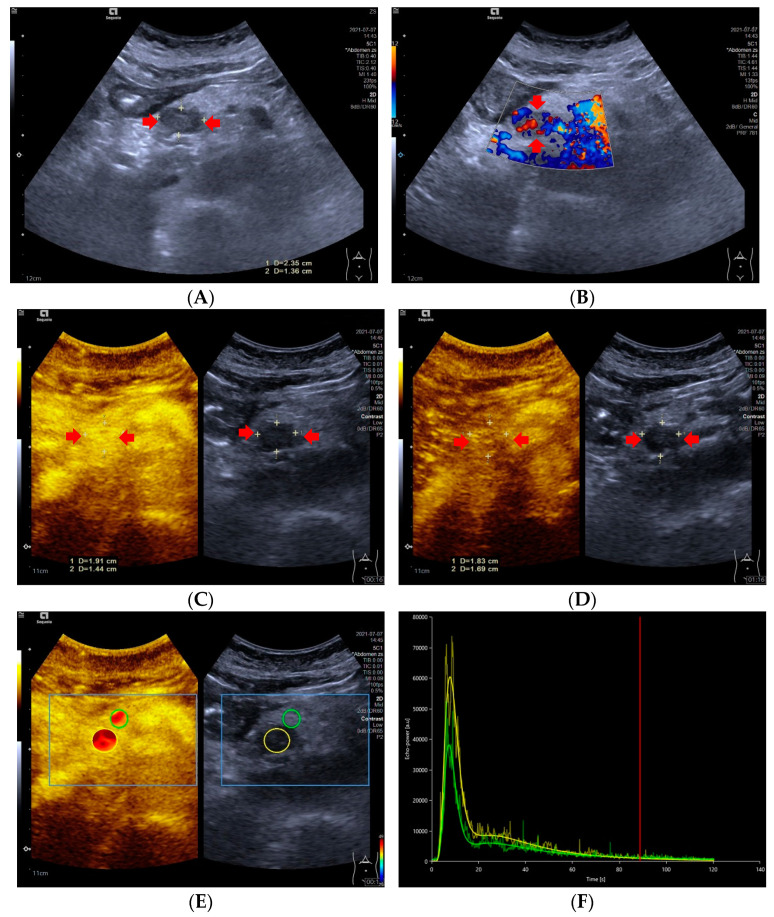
A 59-year-old female with a pNETs G1 lesion (Ki 67 proliferation index of 2%). The pNETs lesion located in pancreatic head was hypoechoic (A) with abundant internal color doppler flow imaging signal (B). After injecting the contrast agent, pNETs lesion showed hyperenhancement in the arterial phase (C) and isoenhancement in the venous phase (D). The pNET’s microvascularization was assessed using VueBox^®^, an external perfusion software. Regions of interest of the pNETs lesion (yellow circle) and surrounding pancreatic parenchyma (green circle) were placed manually (E). The time intensity curve revealed that in the arterial phase, the yellow curve (pNETs lesion) was higher than the green curve (pancreatic parenchyma), and in the venous phase and late phase, the two curves almost completely overlapped (F).
